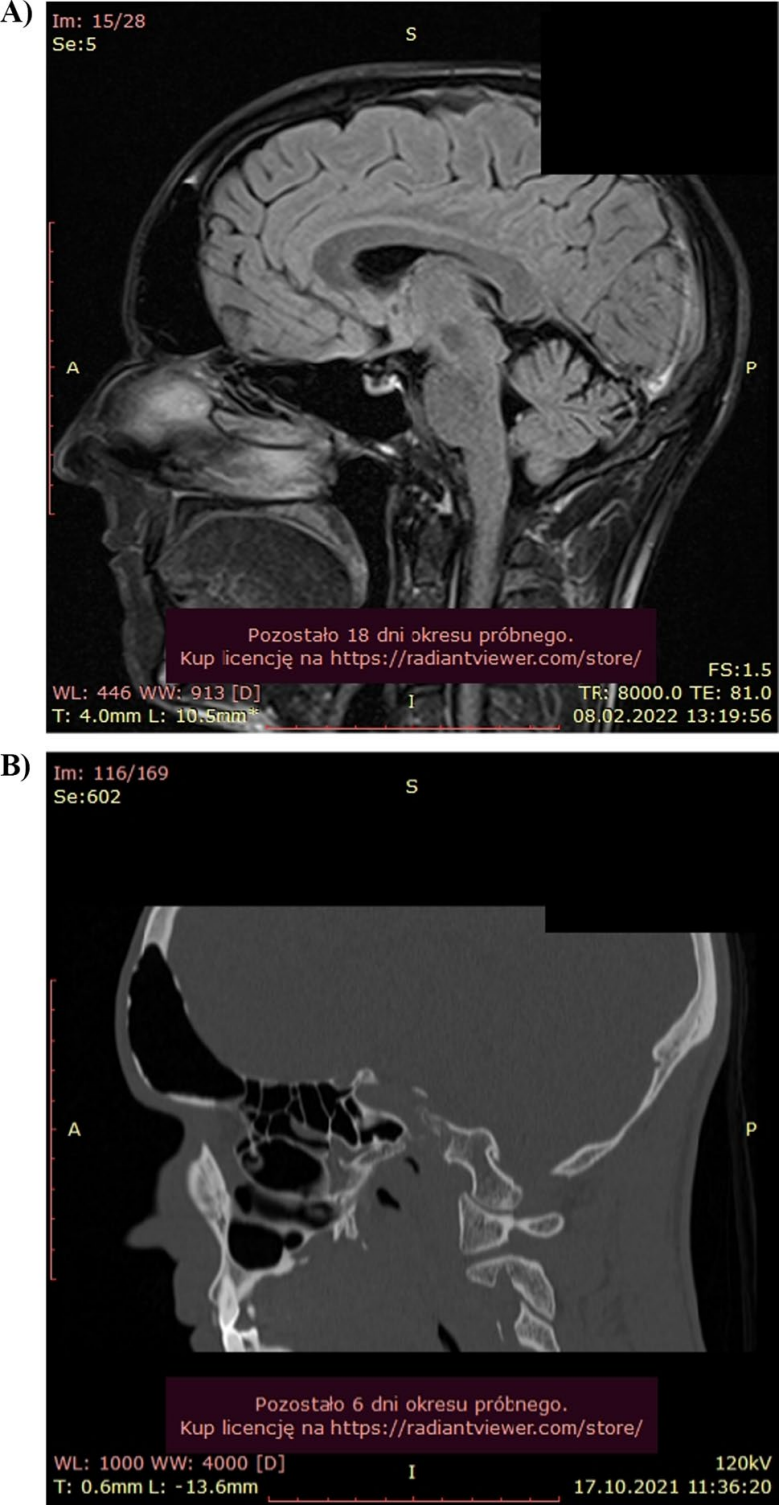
MRI (A) and CT (B) images show the enlargement of the frontal sinus with bone thining
